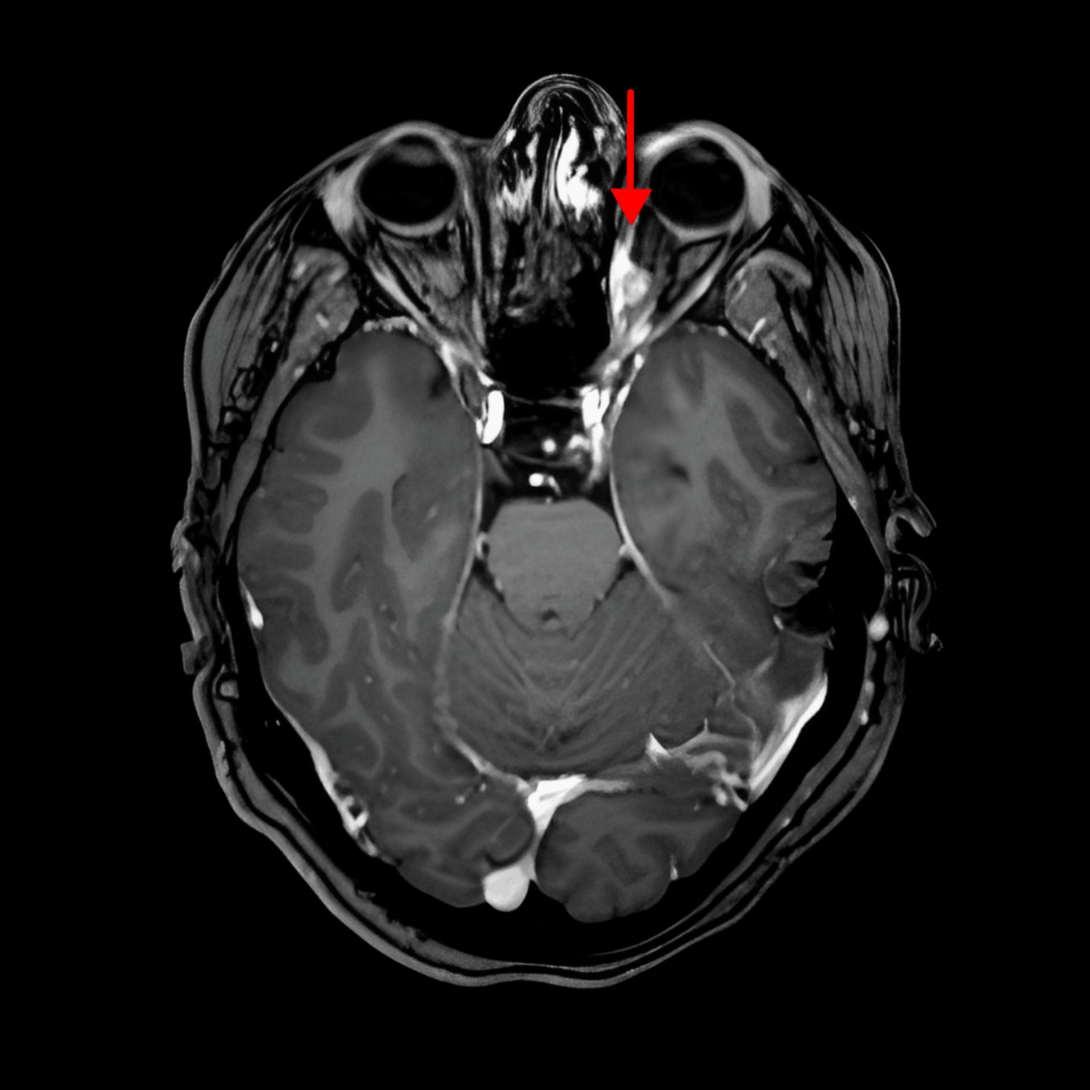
Brain MRI at diagnosis: left intracoronal nodule (9×6 mm) along the medial rectus muscle on the left side MRI: magnetic resonance imaging
